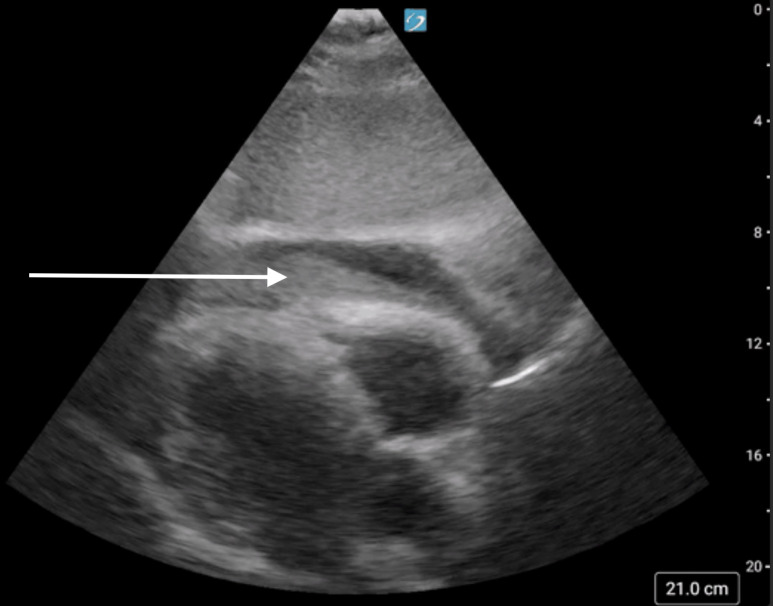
Cardiac point of care ultrasound (POCUS). Subxiphoid view of the heart with large pericardial effusion containing clotted blood (white arrow).
